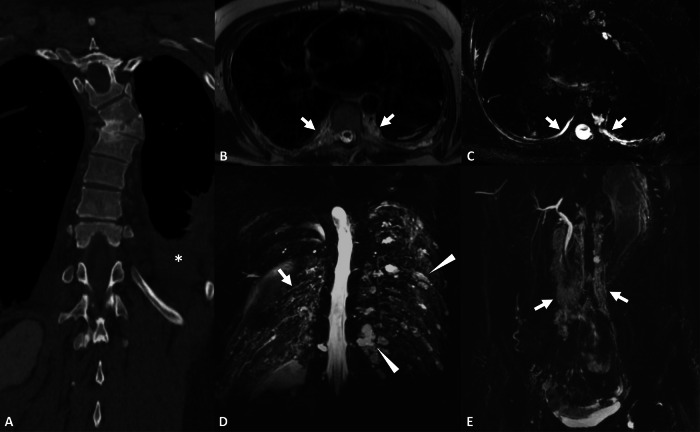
Vertebral compression fracture in a 38-year-old female patient with a history of unexplained chylothorax, revealing GLA with bone destruction (Gorham–Stout disease). A Noncontrast CT, coronal view. Osteolysis of the right half of the Th7 vertebral body, with pseudo-fracture aspect, responsible for deformity in the coronal plane with lateral inflexion. B T2 TSE, axial view. Paravertebral and intercostal infiltration demonstrating high-intensity signal on T2w images (arrows). C 3D FRFSE T2w, MIP reconstruction (20 mm), axial view. Reveals paravertebral lymphangiectasis (arrows) corresponding to paravertebral infiltration on T2w images. D, E 3D FRFSE T2w, MIP reconstruction (20 mm), coronal view. NEMRL reveals additional intercostal lymphangiectasis (arrow) and intercostal lymphangiomas (arrowheads). More lymphatic anomalies are identified below the diaphragm, with retroperitoneal lymphangiectasis (arrows)
